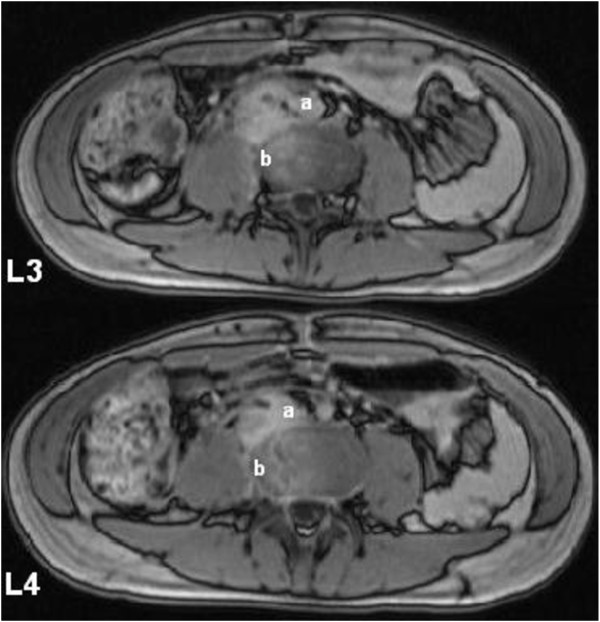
Diagnostic MRI. Contrast MRI demonstrated a small paravertebral collection (a) and osteomyelitis at L III – L IV with areas of bone erosion (b) (T1 weighted images are shown).
